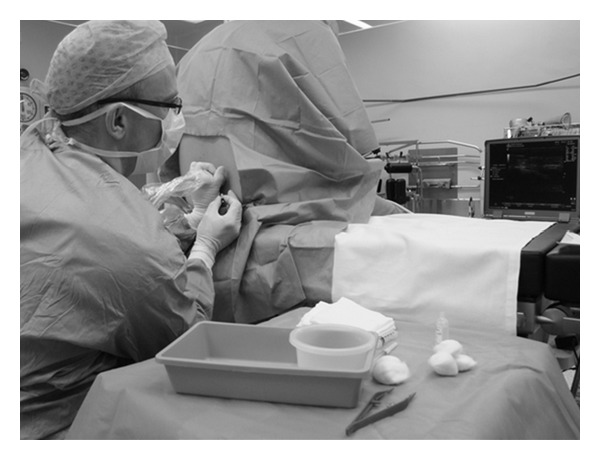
Typical positioning of the operator, ultrasound transducer and the patient during, real-time ultrasound-guided spinal anaesthesia.
